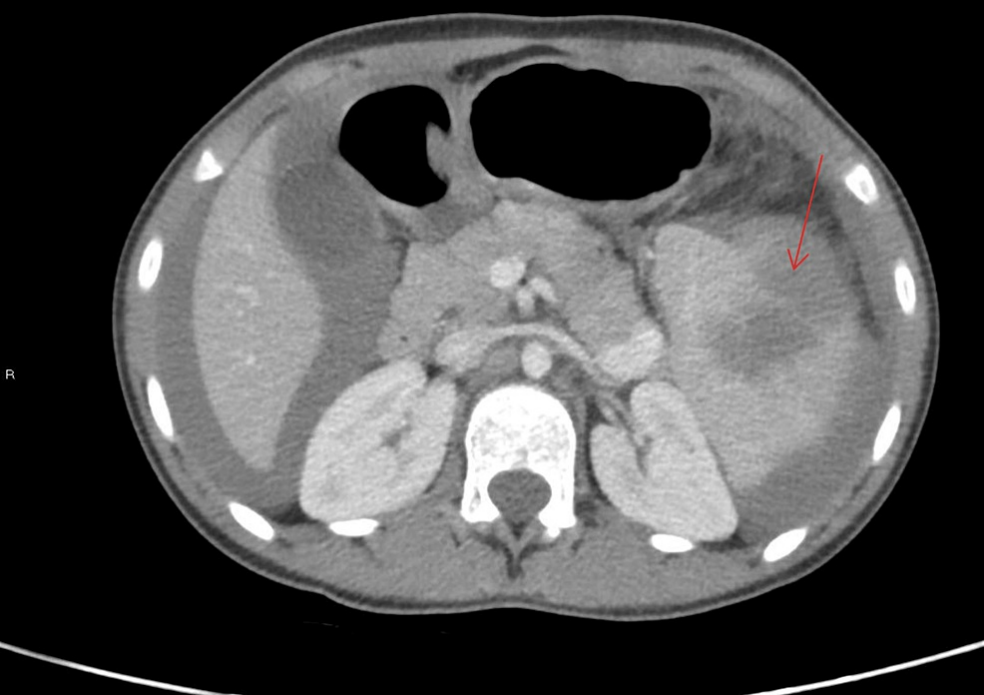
Abdominal CT scan with evidence of splenic rupture and intra-abdominal bleeding (red arrow)
